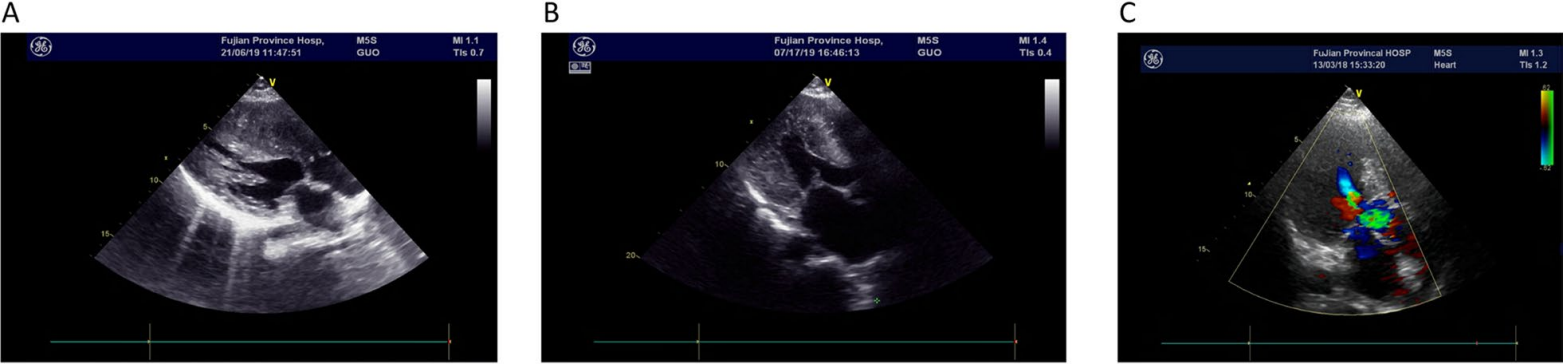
Echocardiography of FHCM patients showed abnormal ventricular septal thickness. a Patient 1, female, 27 years old. Echocardiography showed that the left ventricular myocardium showed asymmetric hypertrophy, and the thickest left ventricular wall was 2.38 cm. b Patient 2, male, 68 years old. Echocardiography showed that the left ventricular myocardium showed asymmetric hypertrophy, and the thickest left ventricular wall was 2.18 cm. c Patient 3, female, 84 years old. Echocardiography showed that the left ventricular myocardium showed asymmetric hypertrophy, and the thickest left ventricular wall was 3.36 cm
